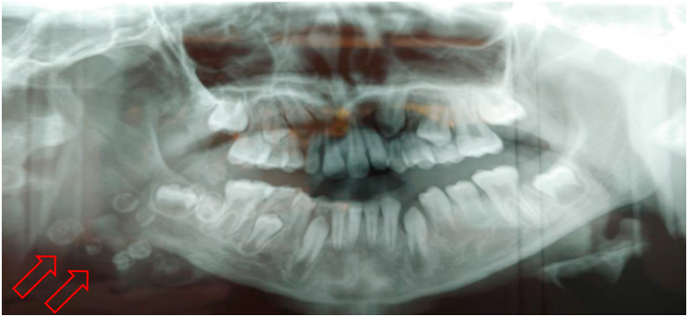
X-ray showing radiopaque images (phleboliths) in the right mandible.
